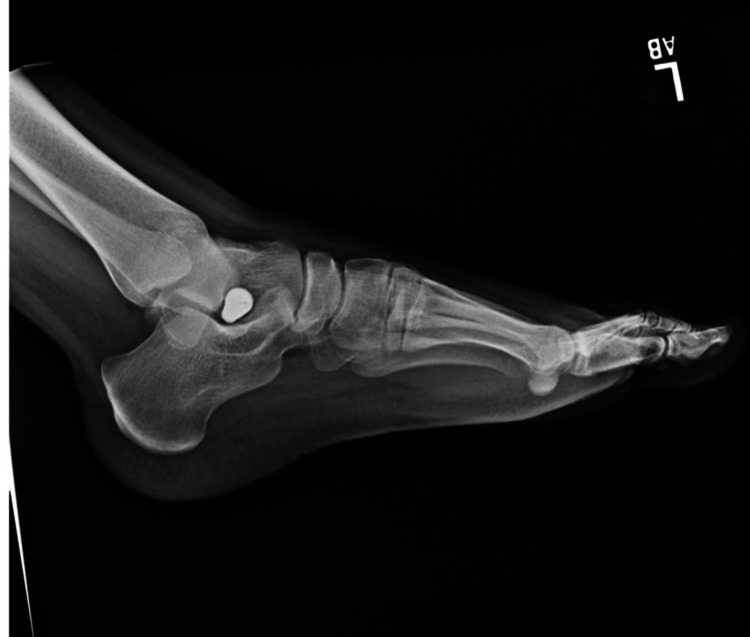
Lateral postoperative left foot weight-bearing X-ray.
